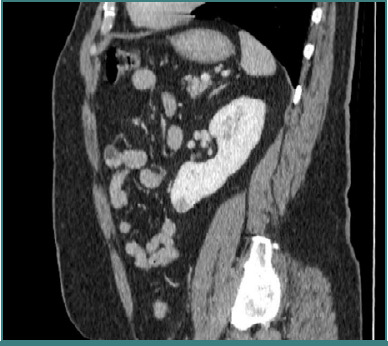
Computed tomography image of a small renal tumor at the level of the lower pole of the left kidney – a robotic partial nephrectomy was performed
